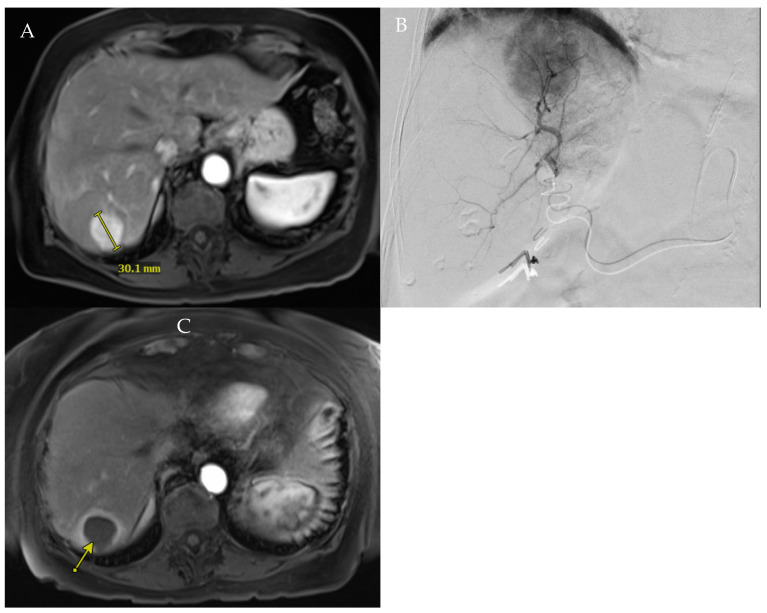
(A). Baseline MRI showing hyper-enhanced 3 cm tumor in segment 7. (B). Angiography of the segment 7 branch of the posterior right hepatic artery confirmed tumor perfusion. (C). Patient underwent RADSEG, with 1-month follow-up showing complete response of treated tumor (arrow).
